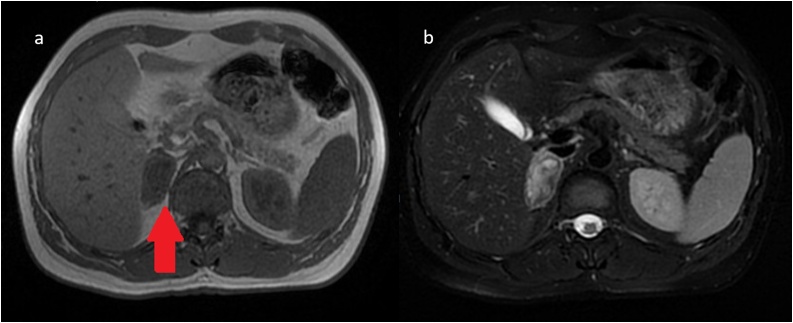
7-day follow-up MRI shows hypointense enlargement of the right adrenal (arrow) in T1 and an anomeogenous gland with hyperintense elements possibly haemorrhagic in diffuse weighted imaging (DWI).
